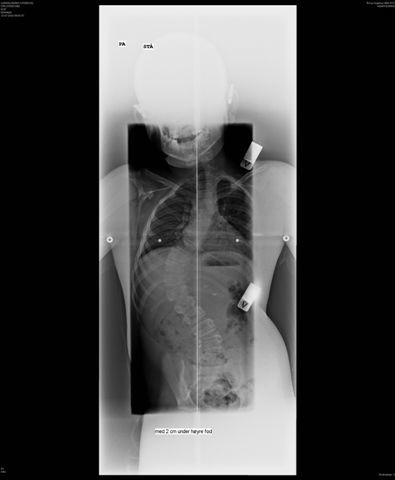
Scoliosis: radiograph showing our patient’s scoliosis.
